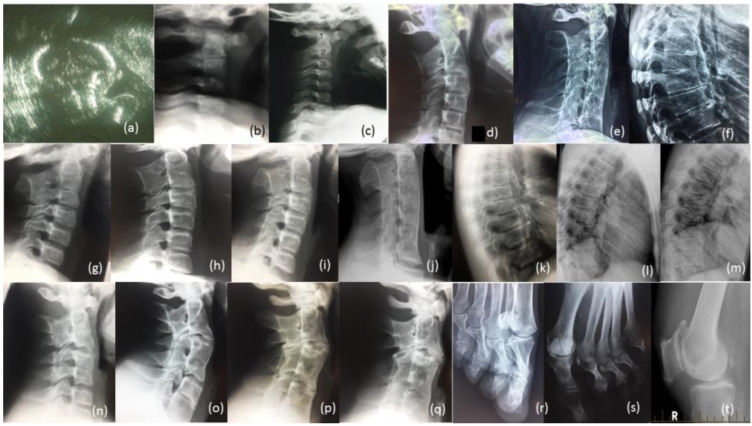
Radiological review of family. Legend: (a) Prenatal ultrasound of cervical spine of proband 36 Weeks; (b) Radiographs of cervical spine of proband age 10 weeks, (c) 12 months (d) 13 years (e) 27 years; (f) Radiograph of thoracic spine of proband age 27 years; (g) Radiograph of cervical spine of brother IV-10 age 7 years, (h) age 14 years, (i) age 17 years, (j) age 26 years; (k) Radiograph of thoracic spine of IV-10 age 17 years, (l) age 26 years, (m) father III-6; (n) Radiograph of cervical spine of brother IV-9 age 12 years, (o) age 19 years, (p) cousin IV-5 age 17 years, (q) age 24 years; (r) Bilateral deviation of the proximal phalanges cousin IV, (s) aunty III-2, (t) Bilateral spurs on patella Aunty III-5 age 65 years.
